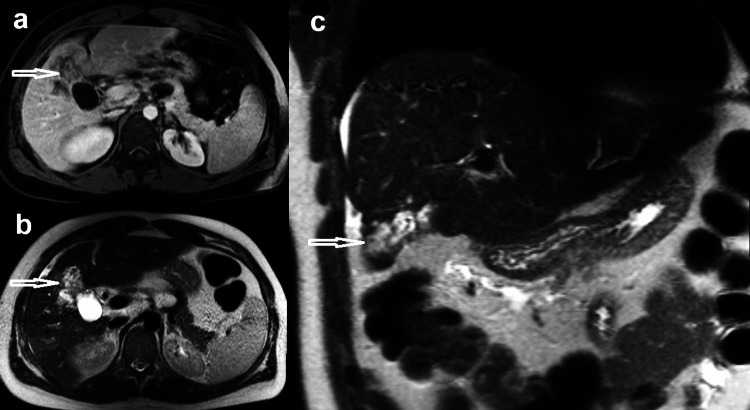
Selected images of magnetic resonance imaging showing a focal area of parenchymal atrophy with tubular hypointense area (arrow) on axial T1-weighted image (a), and hyperintense area (arrow) on axial T2-weighted image (b) in segment IV/V adjacent to the GB with mild enhancement (arrow) and retracted adjacent liver capsule appear on coronal section (c)
